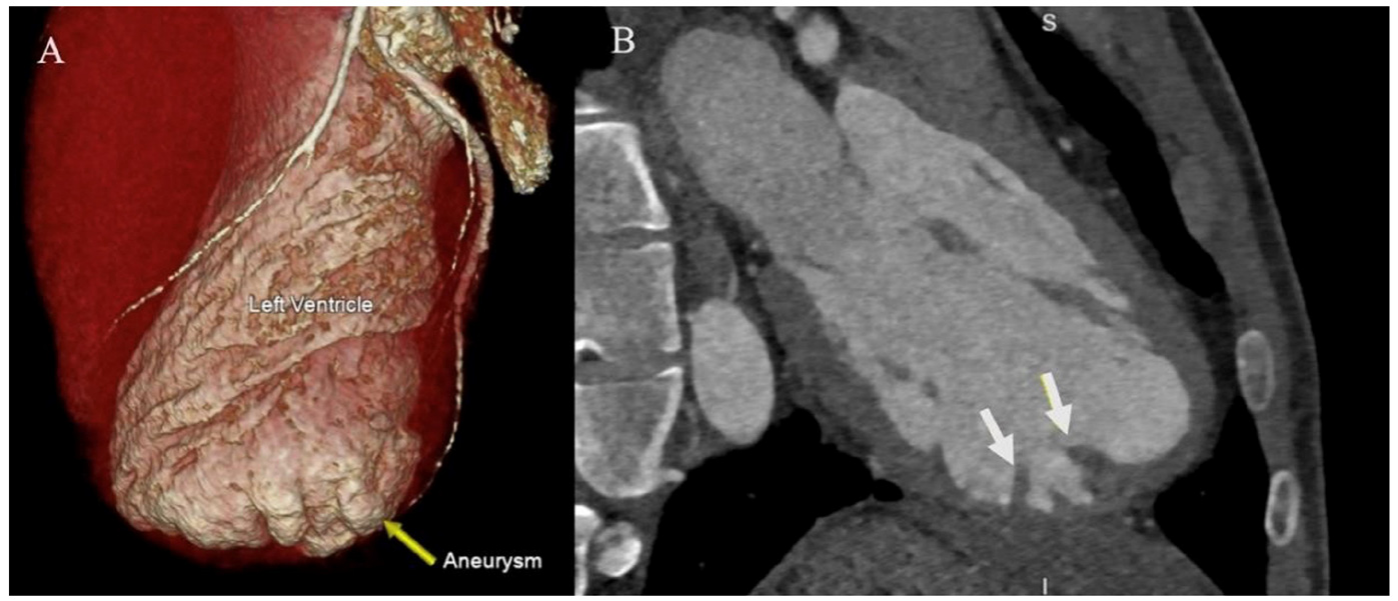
Cardiac CT imaging revealed an isolated apical LV aneurysm (yellow arrow) with apical trabeculations (white arrows).
